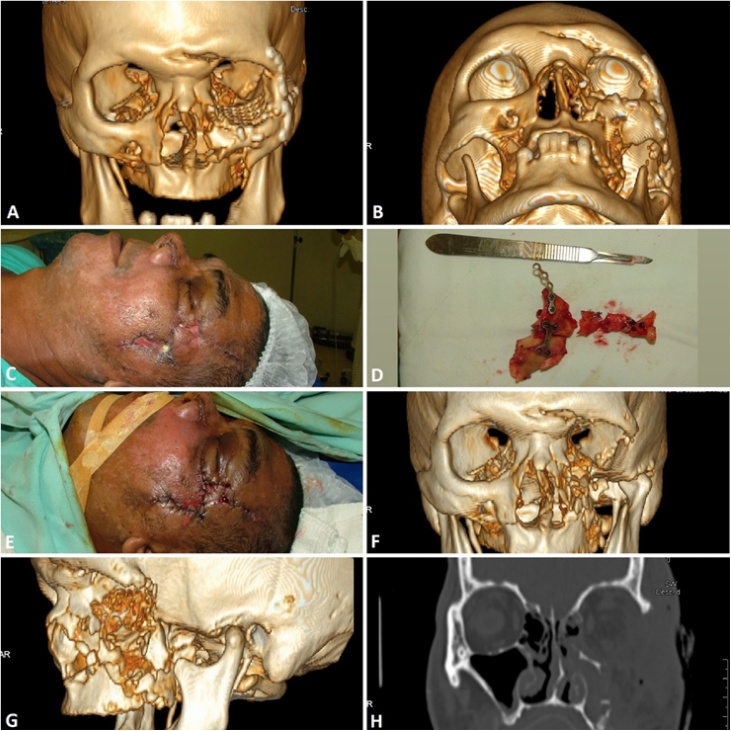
Immediate postoperative 3d reconstructive CT scan. Showing orbitozygomatic (A) and zygomatic arch (B) reconstruction. Clinical view of post-operative infection (C), removed plate and screws with devitalized bone fragments (D), and final appearance after second surgery (E). Post-operative CT scan (F-G). Frontal (F) and lateral (G) 3D reconstruction and coronal (H) cut of CT scan view showing the massive orbital defect.
